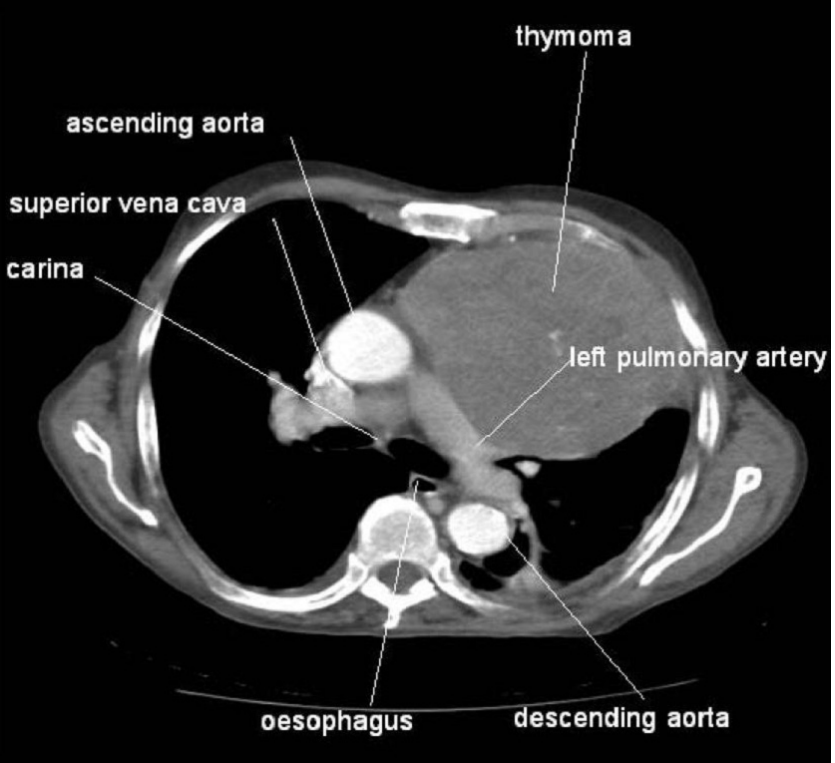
Computed tomography image of thymoma. Figure shows image at level of carina, with large left-sided thymoma. Major mediastinal structures are labelled for orientation purposes.
